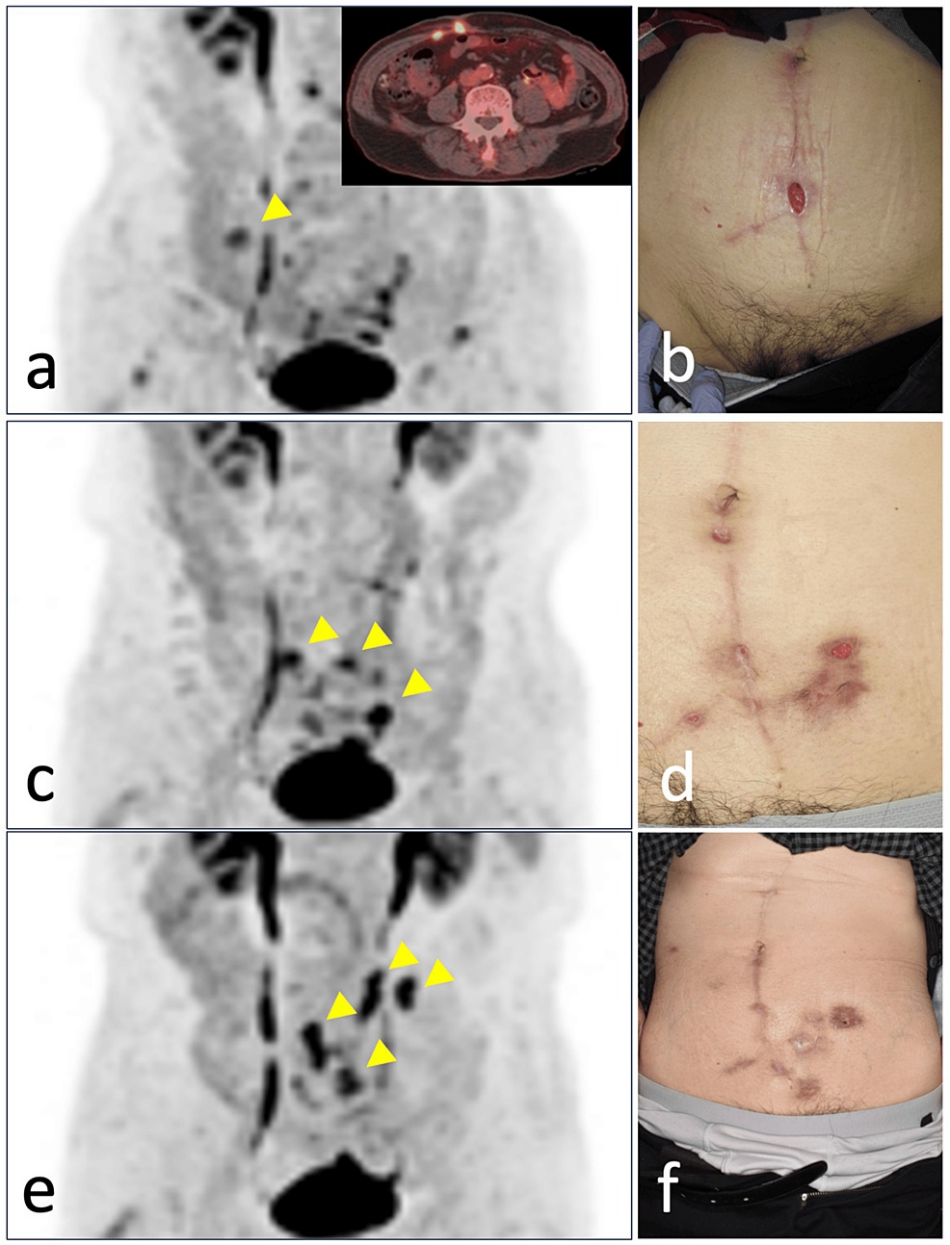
Assessment of infection locus migration via PET-CT (continuation of case 1). PET findings at six months (a), 12 months (c), and 18 months (e) post-maxillectomy for oral cancer. Arrowheads indicate regions where inflammation was suspected on PET. Clinical photographs of the abdominal wall at each respective time point are shown (b, d, f).
